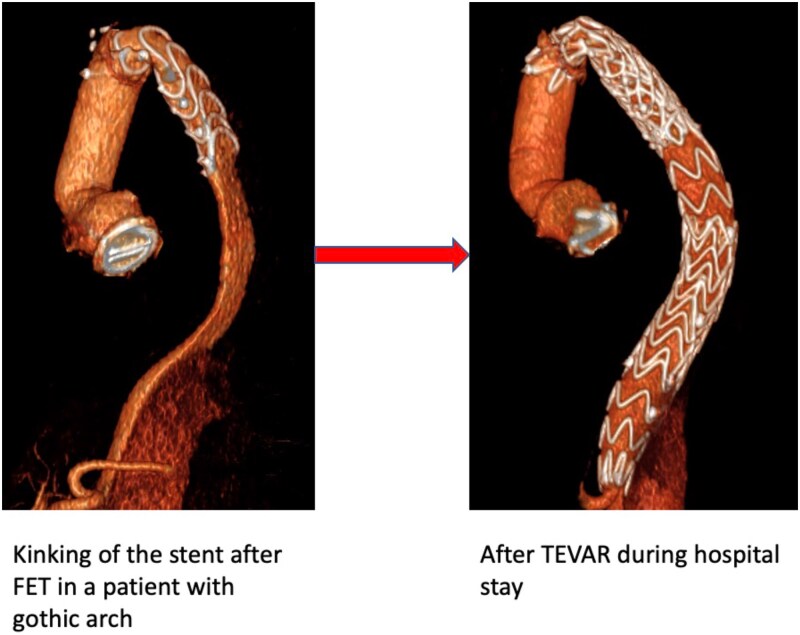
Computed tomography images showing a kinked stent graft after FET in a gothic aortic arch, with subsequent TEVAR extension to restore flow. Example of Stent Kinking After FET in a Patient with Gothic Arch, Treated with In-hospital TEVAR Extension for Visceral Malperfusion
